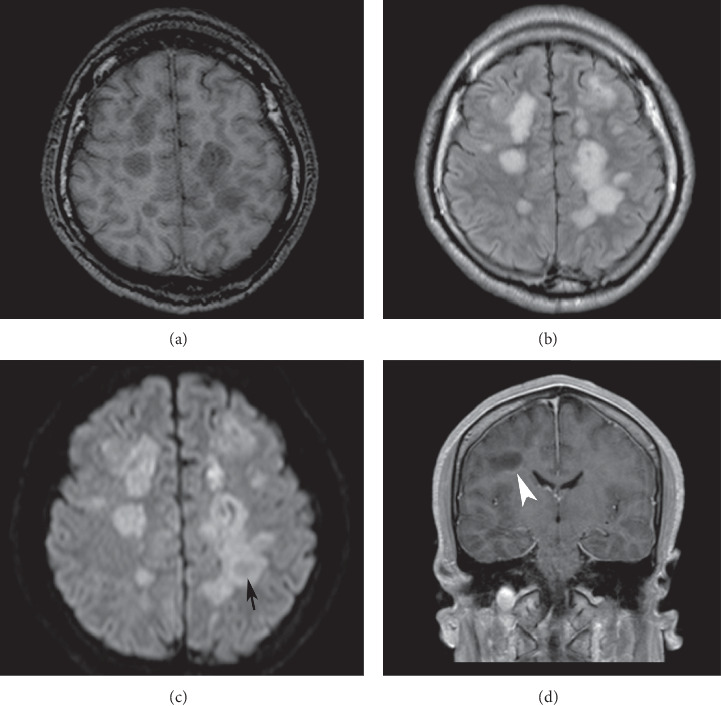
Case report's neuroimages. Axial and coronal MRI with T1 (a), FLAIR (b), diffusion (c), and contrast-enhanced T1, (d) weighted images show various predominant white matter (nodular and confluent) lesions hypointense on T1, hyperintense on T2 (not shown), and FLAIR. With diffusion restriction, some of them had fewer signals at the center (black arrow). After gadolinium administration, most of the lesions have mild incomplete annular enhancement (white arrowhead). MRI : magnetic resonance imaging; FLAIR : fluid-attenuated inversion recovery.
